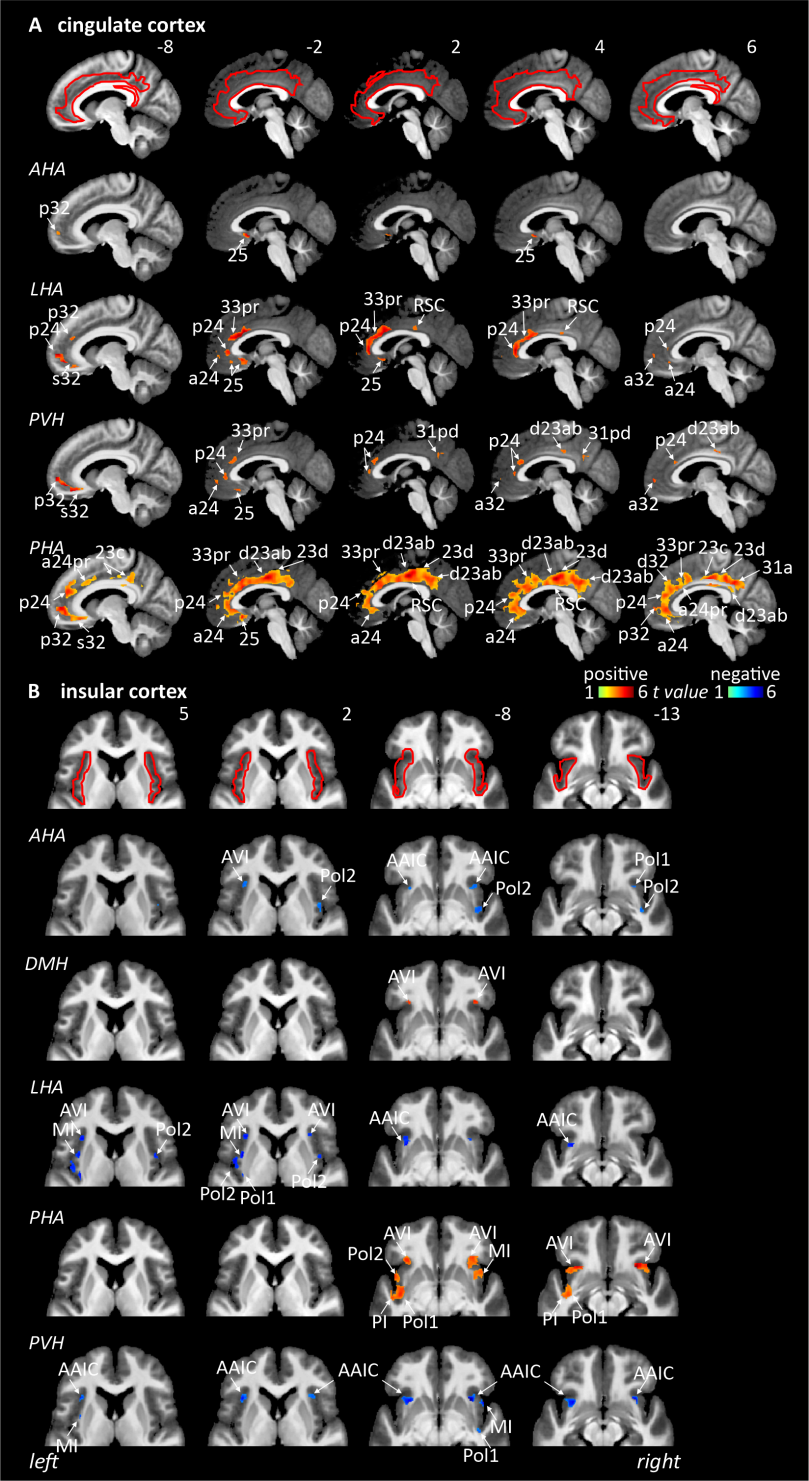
Resting connectivity between six hypothalamic seed regions and the cingulate and insular cortices (n = 150). Areas of significant positive (hot colour scale) and negative (cool colour scale) connectivity overlaid onto a series of sagittal and axial mean T1-weighted anatomical images. Slice locations in Montreal Neurological Institute Space are indicated at the top right of each slice. Red outlines indicating region of interest masked applied for the analysis. (A) Cingulate cortex. (B) Insula. For abbreviations, see table.
